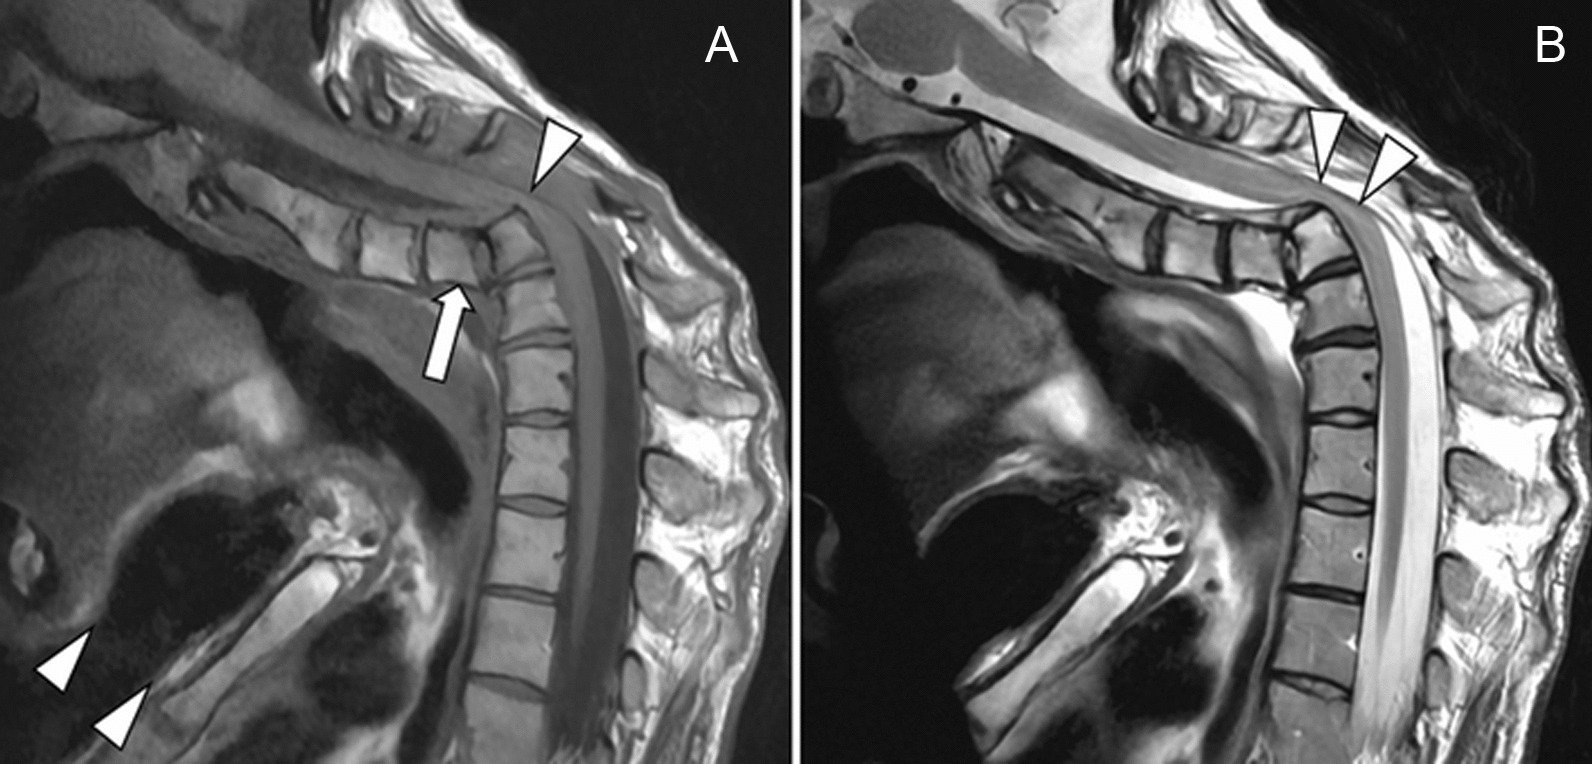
Initial magnetic resonance imaging: sagittal T1 (A) and T2 (B) weighted images showing the spondyloptosis of C4 (arrow) at the anterior aspect of the C5 body, and severe canal stenosis at the level of the posterosuperior angle of the C5 vertebral body (arrowhead in A). Note the close proximity between the chin and the sternum (less than 2 cm, arrowheads in A). Compressive myelopathy with high signal intensity of the spinal cord is evident on the T2 weighted image, involving the C4–C6 segment (arrowheads in B). The residual anteroposterior diameter of thecal sac was less than 5 mm
